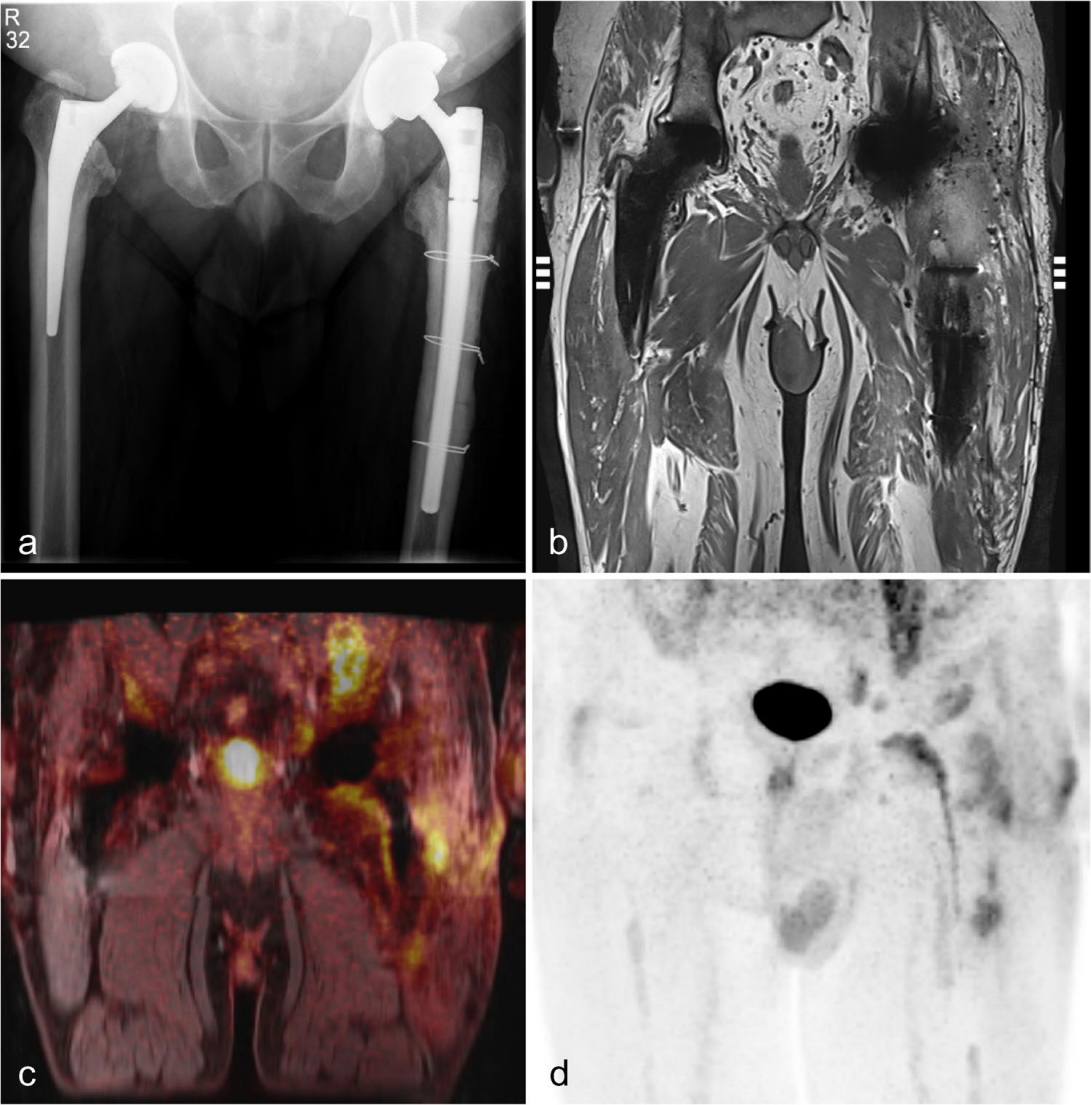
A X-ray of a 55-year-old man with bilateral hip prostheses and left-sided cerclage. B Coronal T1-weighted magnetic resonance imaging (MRI) shows slight metal artefacts in both prostheses and left-sided fluid collections around the neck of the prosthesis. Fused.^18^F-FDG-PET/MRI (C) and the maximum intensity projection image (D) show significant elevated tracer uptake around the prostheses neck, shaft, and soft tissue. In contrast, the left non-infected hip prosthesis reveals a normal tracer pattern. The findings were confirmed by reference standard
